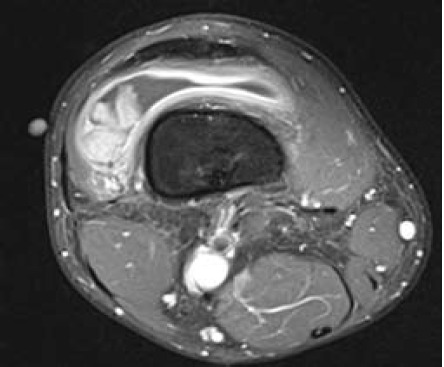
Fig. 1B: Corresponding T2-weighted axial MRI image with fat suppression F1b:
